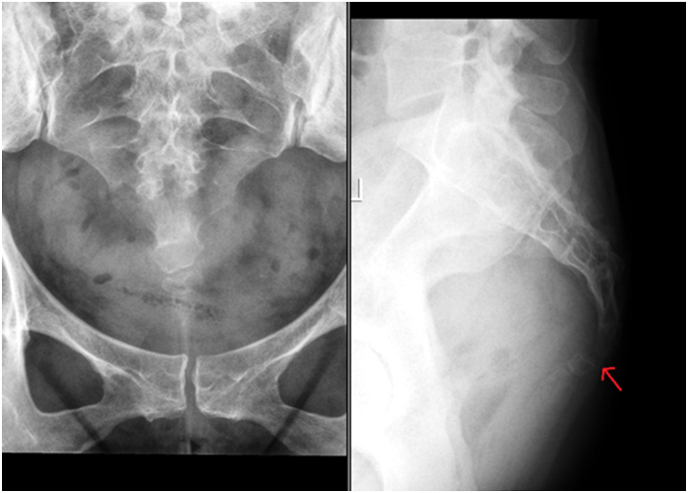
Preoperative coronal and sagittal x-rays demonstrating subluxed sacrococcygeal joint (red arrow).
